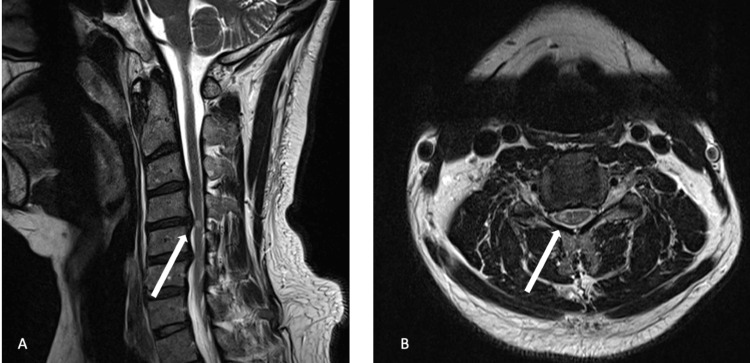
Sagittal (A) and axial (B) T2-weighted MRI of the cervical spine with neutral neck position showing abnormal T2 prolongation involving the right spinal cord at the level of the C4-C5 disc.
